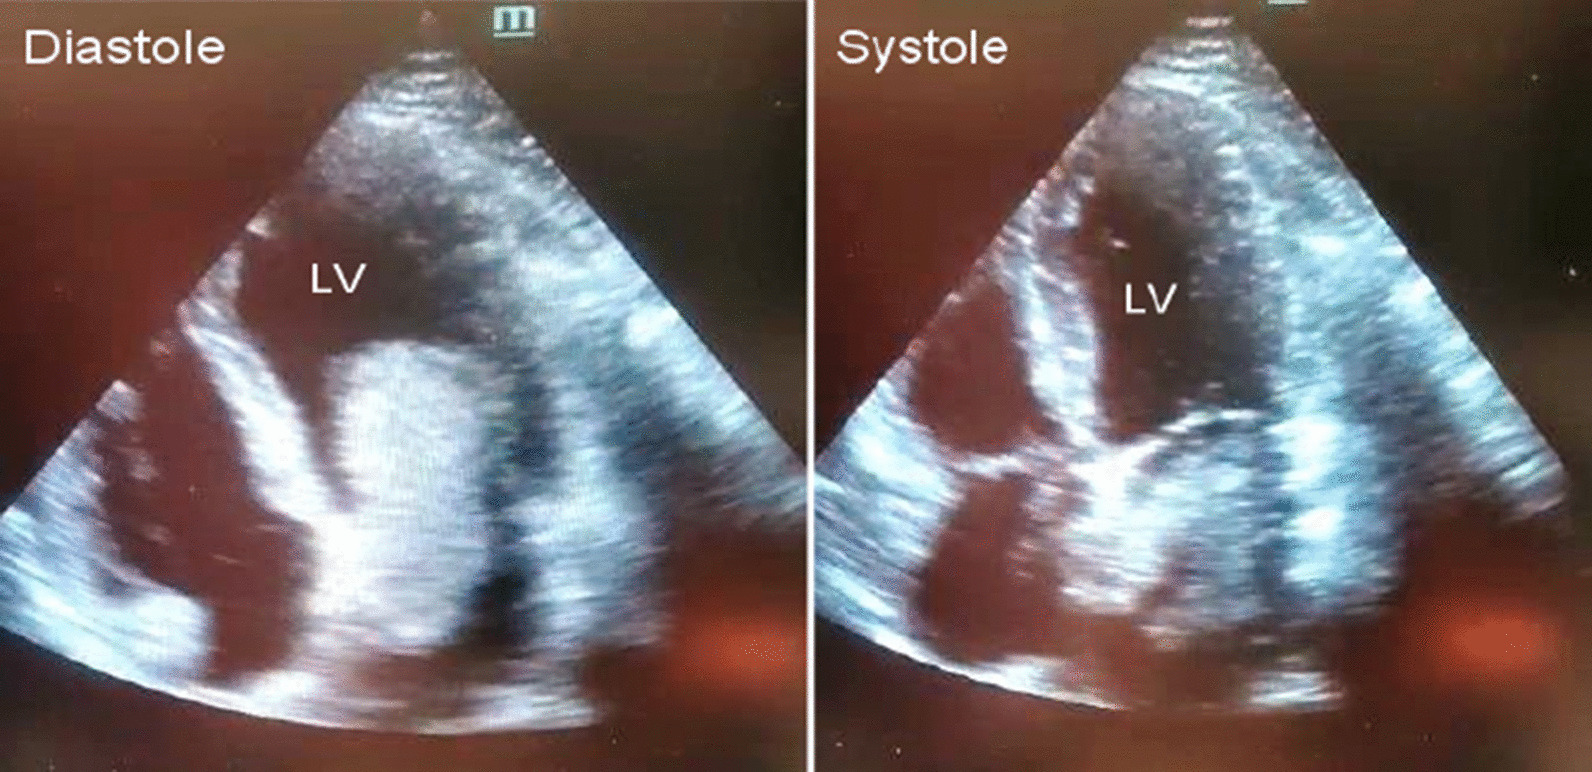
Transthoracic echocardiography shows the left atrial myxoma prolapsing through the mitral valve into the left ventricle during diastole and trapping in the left atrium during systole
